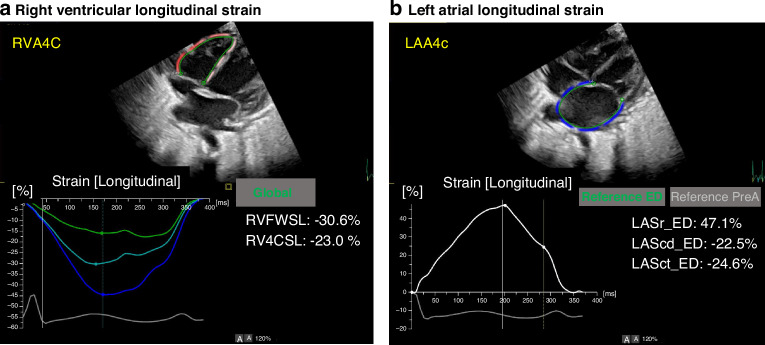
Analysis of right ventricular and left atrial longitudinal strain by speckle-tracking echocardiography. a Right ventricular free wall strain and four-chamber strain obtained from apical four-chamber view (RV A4C). b Left atrial reservoir, conduit, and contraction strain measured from LA apical view using end-diastole as reference. RV right ventricular, LA left atrial, A4C apical four-chamber view, RVFWSL right ventricular free wall longitudinal strain, RV4cSL right ventricular four-chamber longitudinal strain, LASr_ED left atrial reservoir strain with a reference time point at end-diastole, LAScd_ED left atrial conduit strain with a reference time point at end-diastole, LASct_ED left atrial contraction strain with a reference time point at end-diastole.
